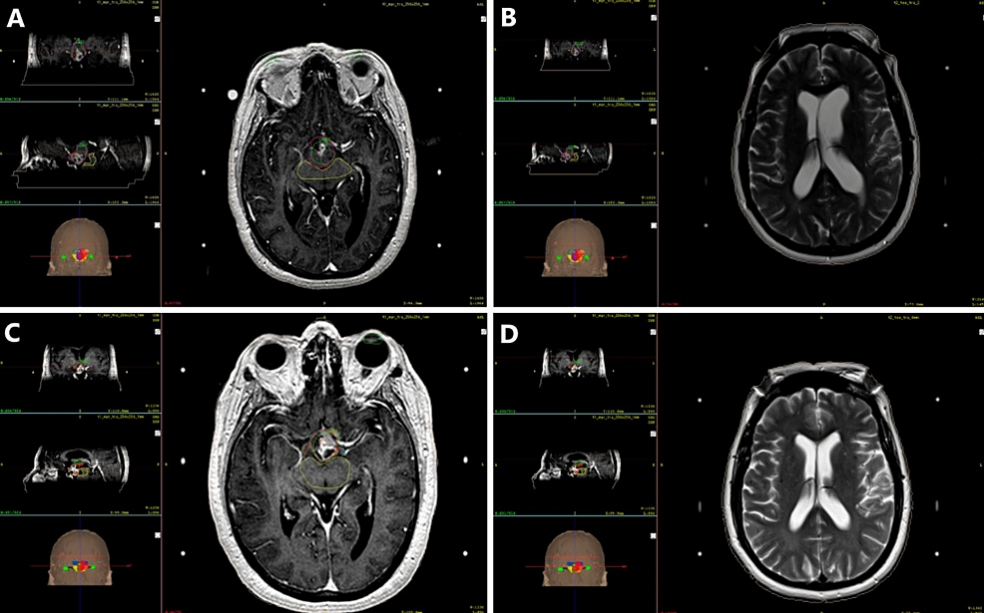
Tumor and hydrocephalus evolution for patient six Top row: prior to SRS. (A) Axial T1 gadolinium-enhanced MRI the day before treatment showed a large hypothalamic glioma. PTV in this plan (7.3 cc) was drawn within the GTV (8 cc). The prescription dose for PTV was 13 Gy at a 50% isodose curve and GTV received a median and maximum prescription dose of 0.5 and 1 Gy, respectively. (B) Axial T2 MRI on the day of treatment, the image shows dilation of the lateral ventricles, the transpendymal edema and a deviation of the septum pellucidum due to hydrocephalus. EVAN’S index at that moment was 0. 34. Bottom row: Second treatment session of two-staged radiosurgery (30 days after). (C) Axial T1 gadolinium-enhanced MRI showed a tumor volume of 4.0 cc, in this case, PTV=GTV. A tumor reduction of 45% was documented compared to the first treatment session. The prescription dose, in this case, was 10.5 Gy at a 50% isodose curve. D) Regarding hydrocephalus, the lateral ventricles were not dilated, the transependymal edema had reabsorbed and septum pellucidum showed no deviation. The patient no longer had symptoms related to hydrocephalus. EVAN’S index was 0.17. PTV: planning target volume; GTV: gross tumor volume; SRS: stereotactic radiosurgery
